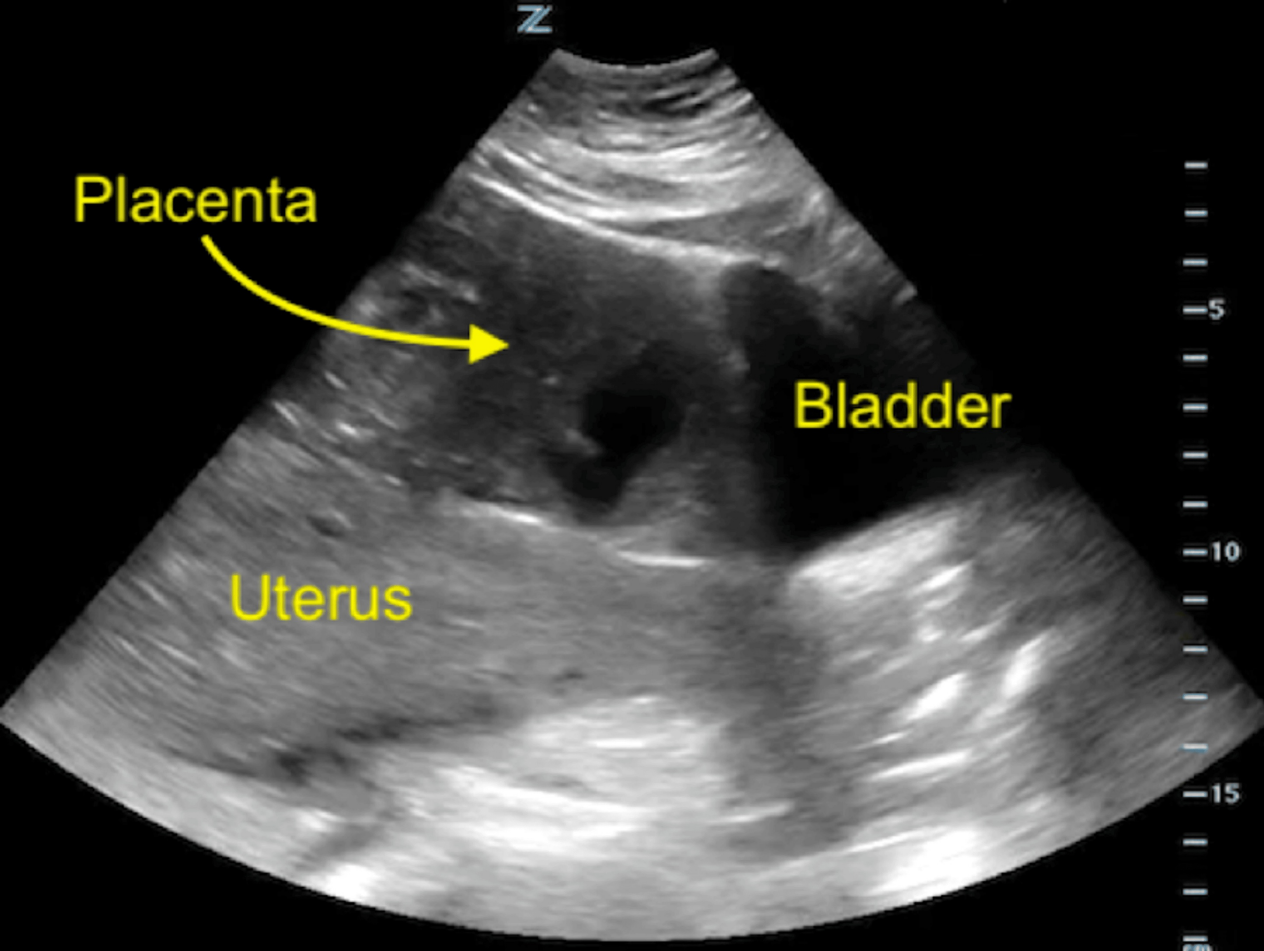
Point-of-care ultrasound in the emergency department showing the uterus and placenta in the sagittal plane
